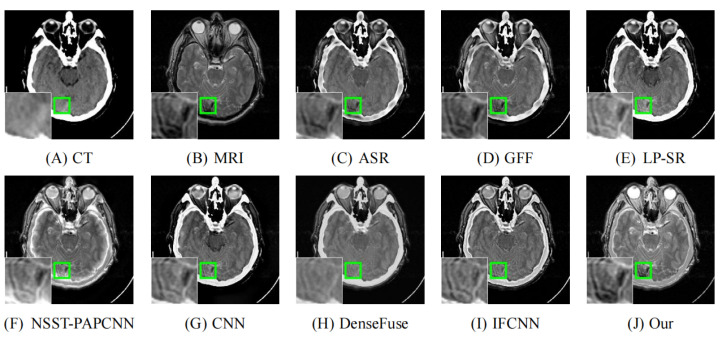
Fusion images of “Acute stroke speech arrest-7” by various methods. From (A) to (J): CT, MRI, ASR, GFF, LP-SR, NSST-PAPCNN, CNN, DenseFuse, IFCNN, Our proposed method.
